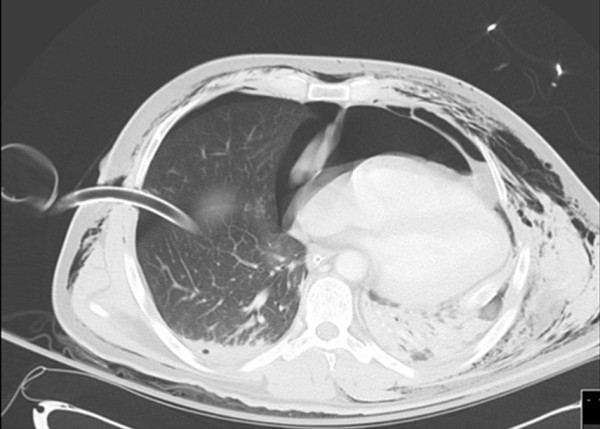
Axial chest CT showing most of the pathology found on the plain radiograph but also bilateral anterior pneumothoraces; large volume anterior pneumopericardium; tube thoracostomies.
